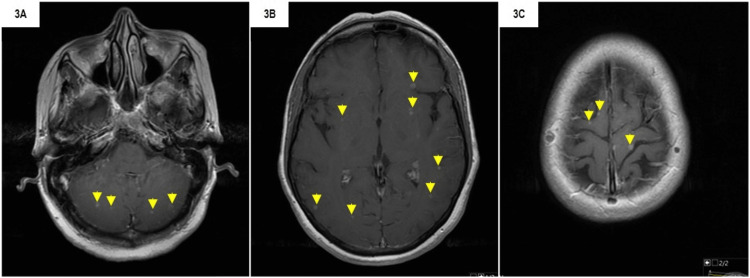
Cerebral MRI showing miliary cerebral metastasis. 3A-3C) multiple hyperintense lesions in T1-weighted with gadolinium and in different axial planes; yellow arrows: example of cerebral lesions
